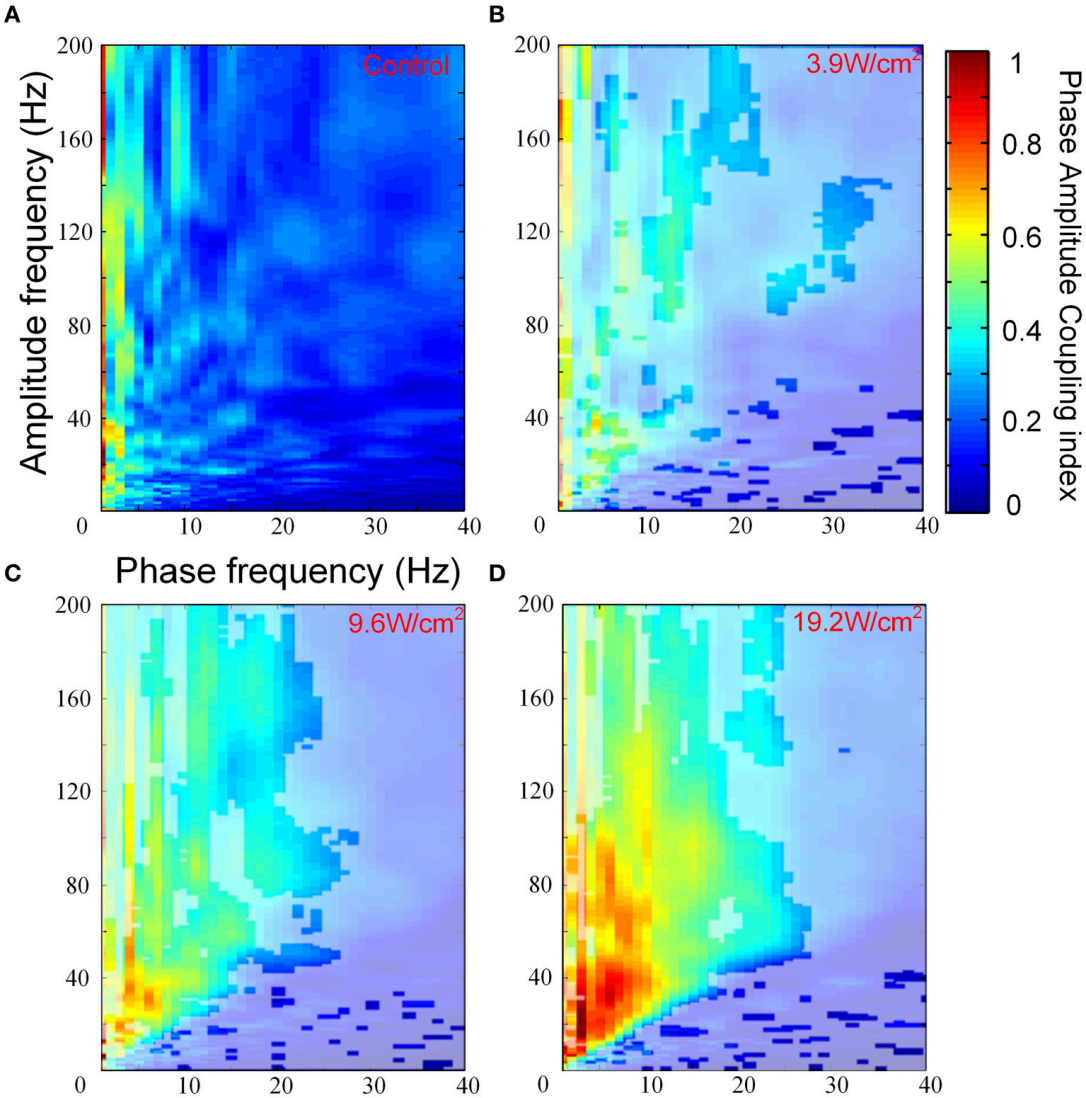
(A) The images of the PACI as a function of the analytic phase (1–40 Hz) and the analytic amplitude (1–200 Hz) for the control (*n* = 6). (B–D) The images of the PACI as a function of the analytic phase (1–40 Hz) and the analytic amplitude (1–200 Hz) for the FUS with different ultrasonic intensities: (B) 3.9 W/cm^2^, (C) 9.6 W/cm^2^, (D) 19.2 W/cm^2^ (*n* = 6).
